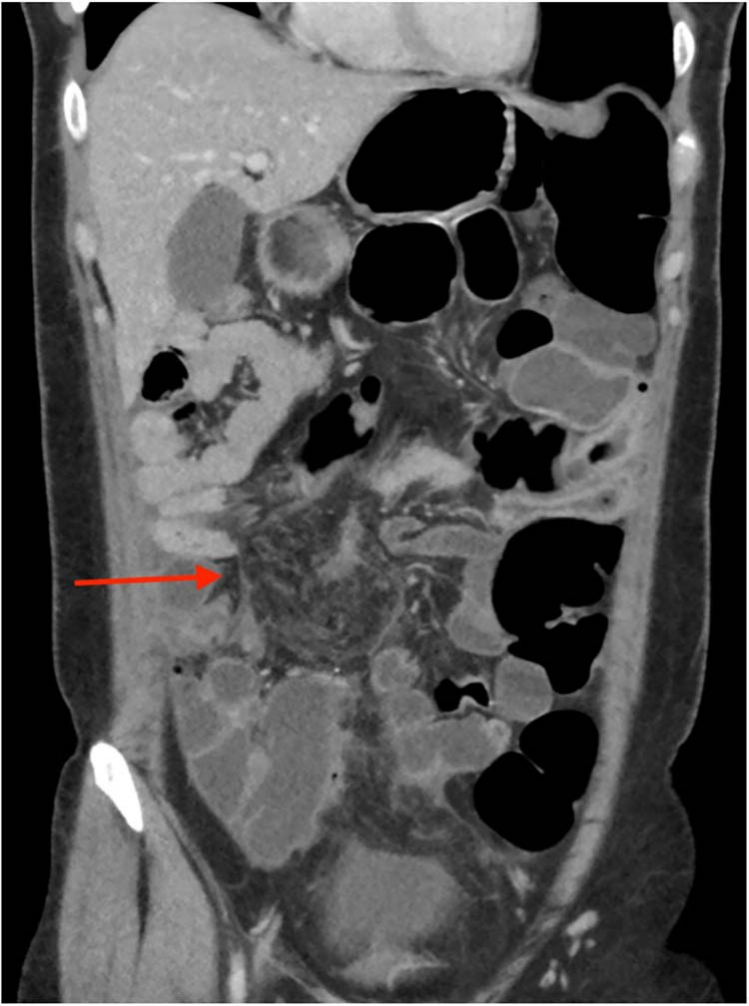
CT abdomen pelvis in 2023 (coronal).
